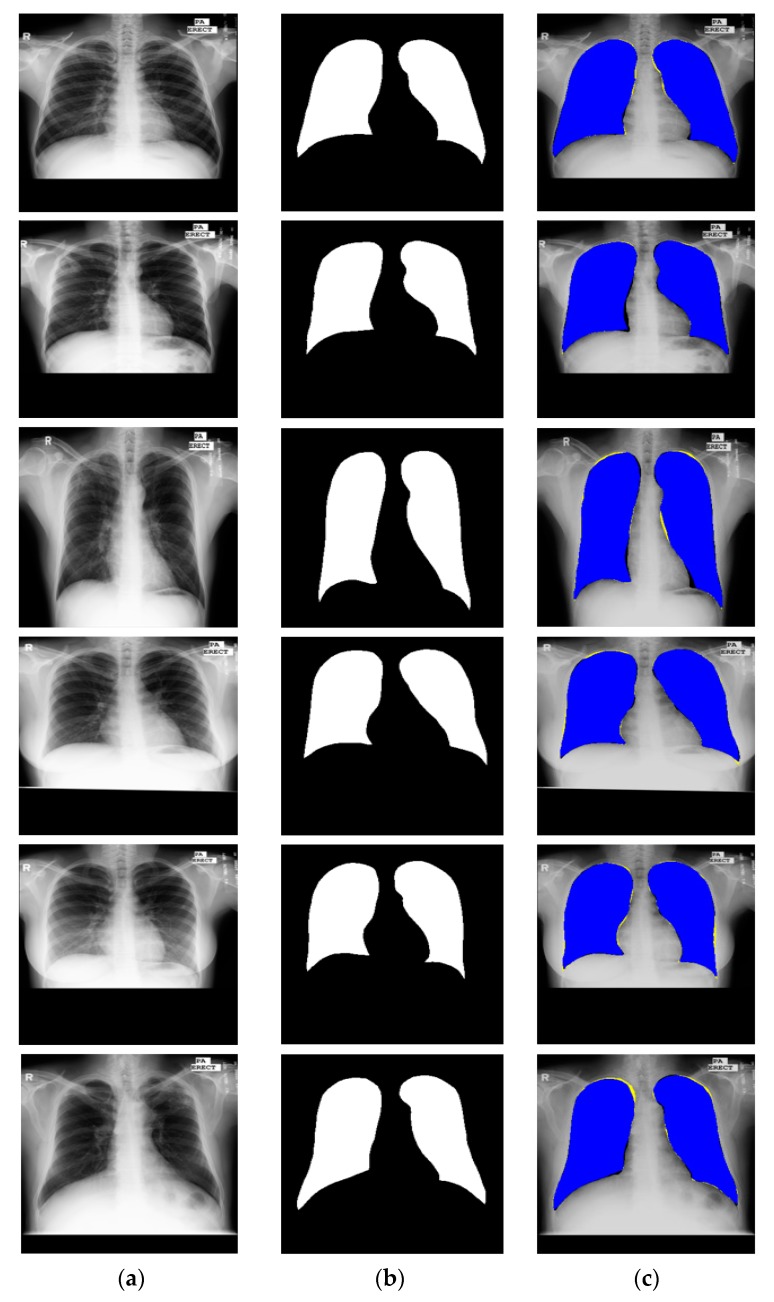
Examples of lung segmentation by X-RayNet for the MC dataset: (a) original image; (b) ground-truth mask; (c) segmented image by X-RayNet (*TP* is presented in blue, *FP* in black, and *FN* in yellow).
